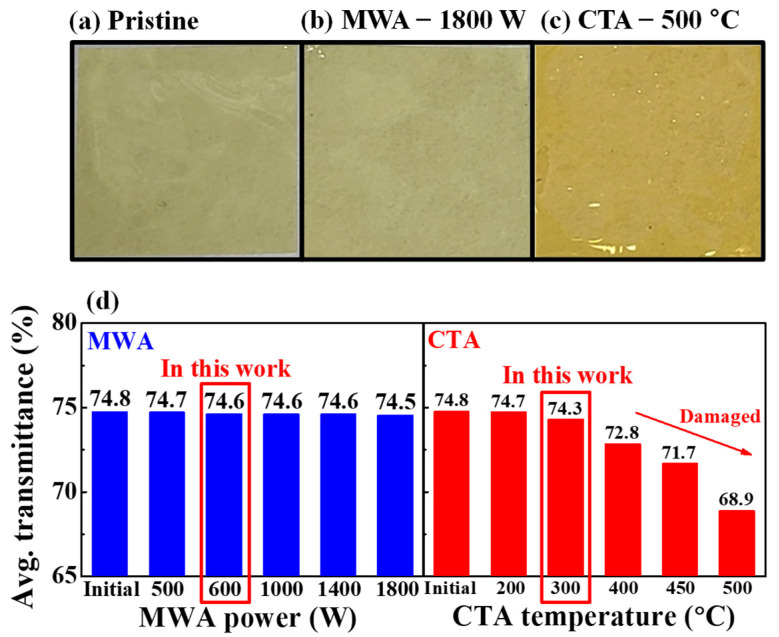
Photographic images of (a) pristine, (b) MWA (1800 W, 2 min) and (c) CTA processed (500 °C, 30 min) PI substrates. (d) Average transmittance of the PI substrates in the visible light region.
